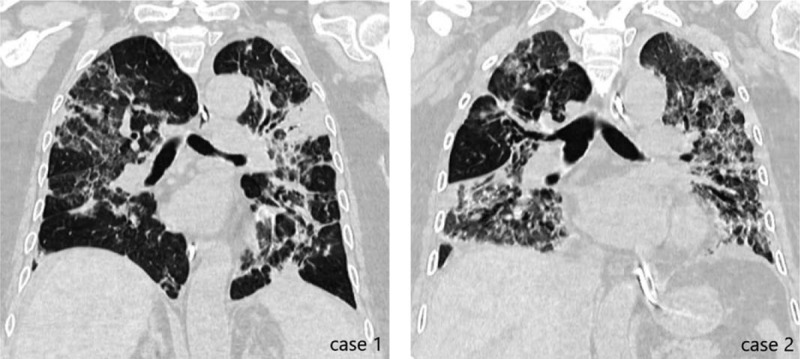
Chest CT scans of case 1 and case 2 when they were extubated for the first time. CT = computed tomography.
